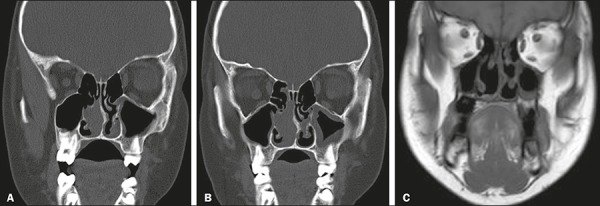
A: Coronal CT scan of sinonasal lymphoma showing a
